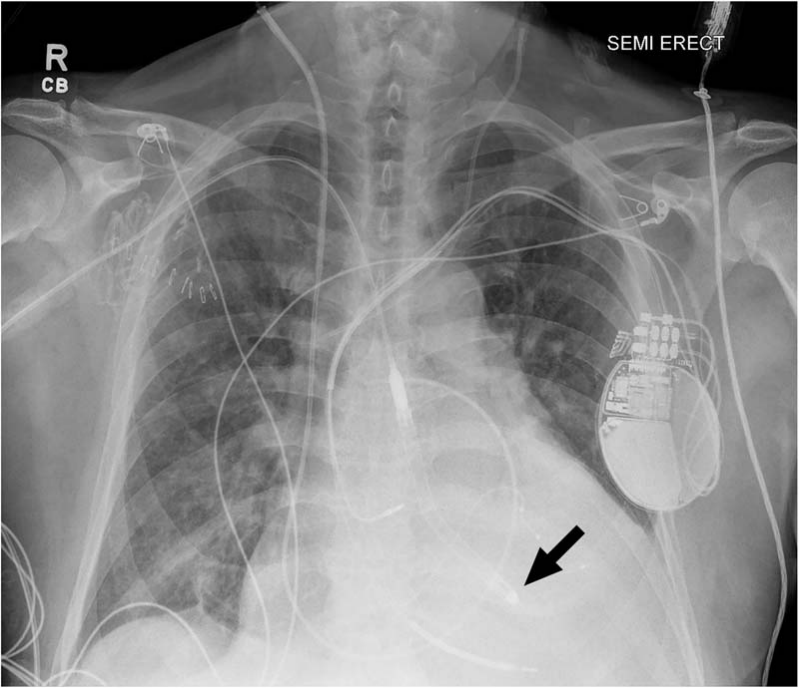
Chest radiograph after Impella 5.5® placement demonstrating adequate placement across the aortic valve into the left ventricle. The arrow indicates the tip of the Impella positioned in the left ventricle.
